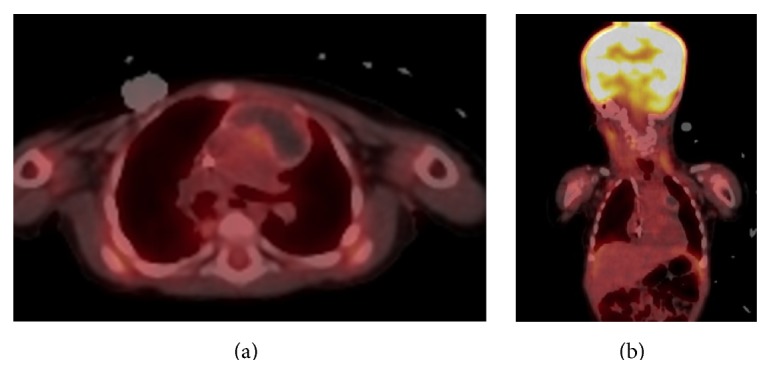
Whole body PET/CT scan showing no significant metabolically active lesions.
